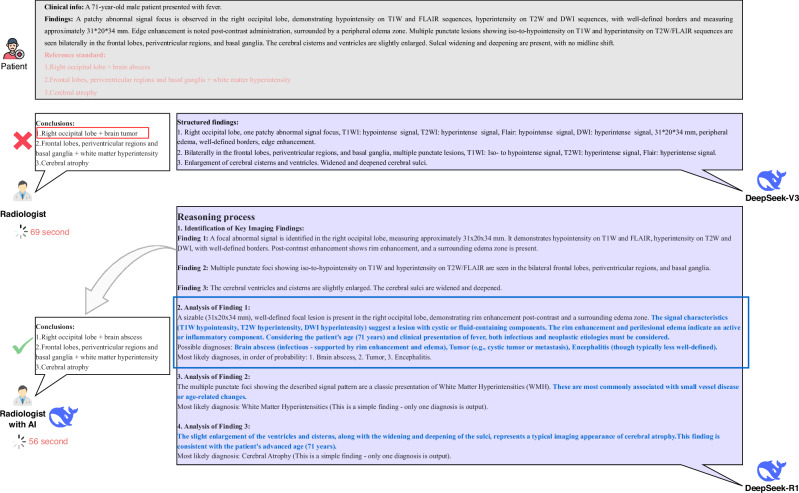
Example of report diagnosis generating process by DeepSeek-R1 in real world reader study. A junior radiologist with 2 years of experience independently interpreted brain MRI report findings and formulated the diagnoses with and without AI assistance. Initially, the radiologist interpreted the brain MRI report findings without any AI support. In this instance, the radiologist misdiagnosed a brain abscess as a brain tumor. After a 6-week washout period, the same radiologist reinterpreted the brain MRI report findings with the aid of DeepSeek’s AI diagnosis and reasoning process. With the assistance of AI, the radiologist correctly diagnosed the case as a brain abscess. The interpreting time reduced from 69 s (without AI) to 56 s (with AI). The blue words indicated the reasoning process for each disease. The red rectangle showed the wrong diagnosis by the junior radiologist without the support of AI. The blue rectangle showed the reasoning process of three differential diagnosis for the wrongly diagnosis by the junior radiologist without the support of AI.
